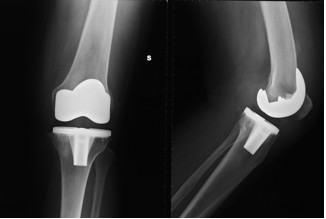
X-rays at six months follow-up examination.
